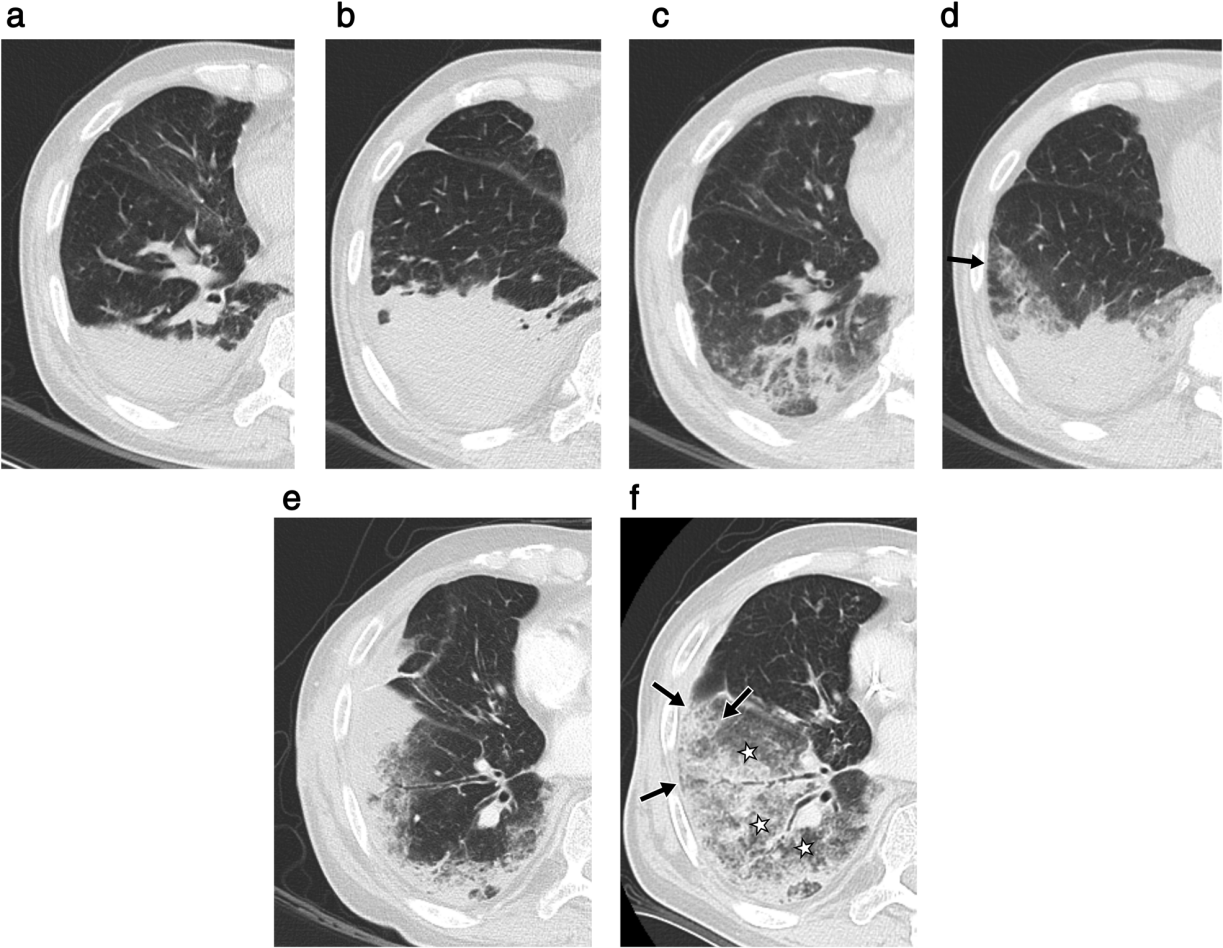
Axial chest CT images (section thickness, 3.0 mm) of a 77‐year‐old male with pneumonic‐type invasive mucinous adenocarcinoma showing two types of spontaneous regression of airspace opacities (SRA). (a, b) Initial chest CT showed consolidation in the dependent portion of right lower lobe. (c) Chest CT scan taken four months after the initial scan showed decreased extent and density of the consolidation. At a slightly caudal level (d) a new patchy ground‐glass opacity (arrow) is seen near the slightly regressed consolidation in the right lower lobe. The patient received antibiotics for three weeks after the initial scan but had not received any anticancer therapy. Although the lesion was later proven to be invasive mucinous adenocarcinoma, regression of airspace opacities without anticancer therapy was observed, and this was termed as SRA. (e) Patchy peripheral consolidation in the right lower lobe was seen on chest CT taken about a year after the initial scan. (f) The patient then received one cycle of cisplatin‐based combination chemotherapy with doxorubicin, and the post‐chemotherapy follow‐up chest CT scan revealed decrease in density and extent of the peripheral consolidation (black arrow), whilst also showing new areas of ground‐glass opacity (stars). This pattern of change after anticancer therapy was also considered to be SRA, because increase or decrease in extent of airspace opacities appears to occur regardless of the use of anticancer therapy.
